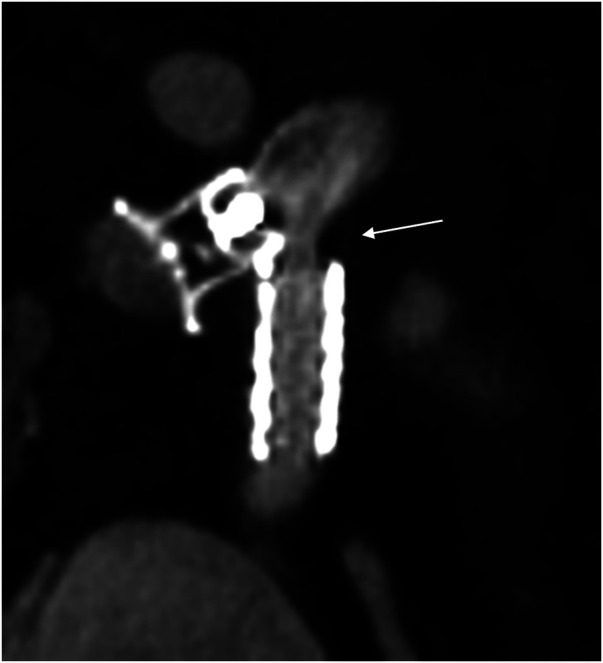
Unenhanced CT image of the ADO I® 10/8 mm closing the distal innominate vein and the covered stent (gore viabahn® 7/19 mm) in the fenestration with proximal stenosis of the fenestration (arrow).
